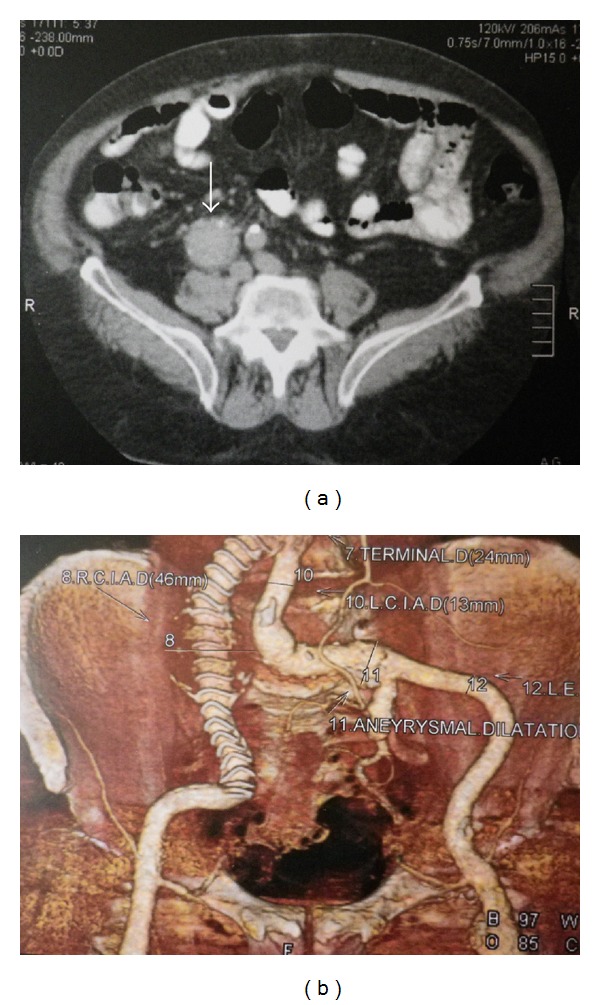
(a) Preoperative contrast-enhanced abdominal CT showing severe aneurysm of the RCIA with diameter 4.5 × 4.7 cm. (b) Contrast-enhanced CT scan reconstruction at 18 months showing patency of the endograft, complete aneurysm exclusion without any endoleak.
